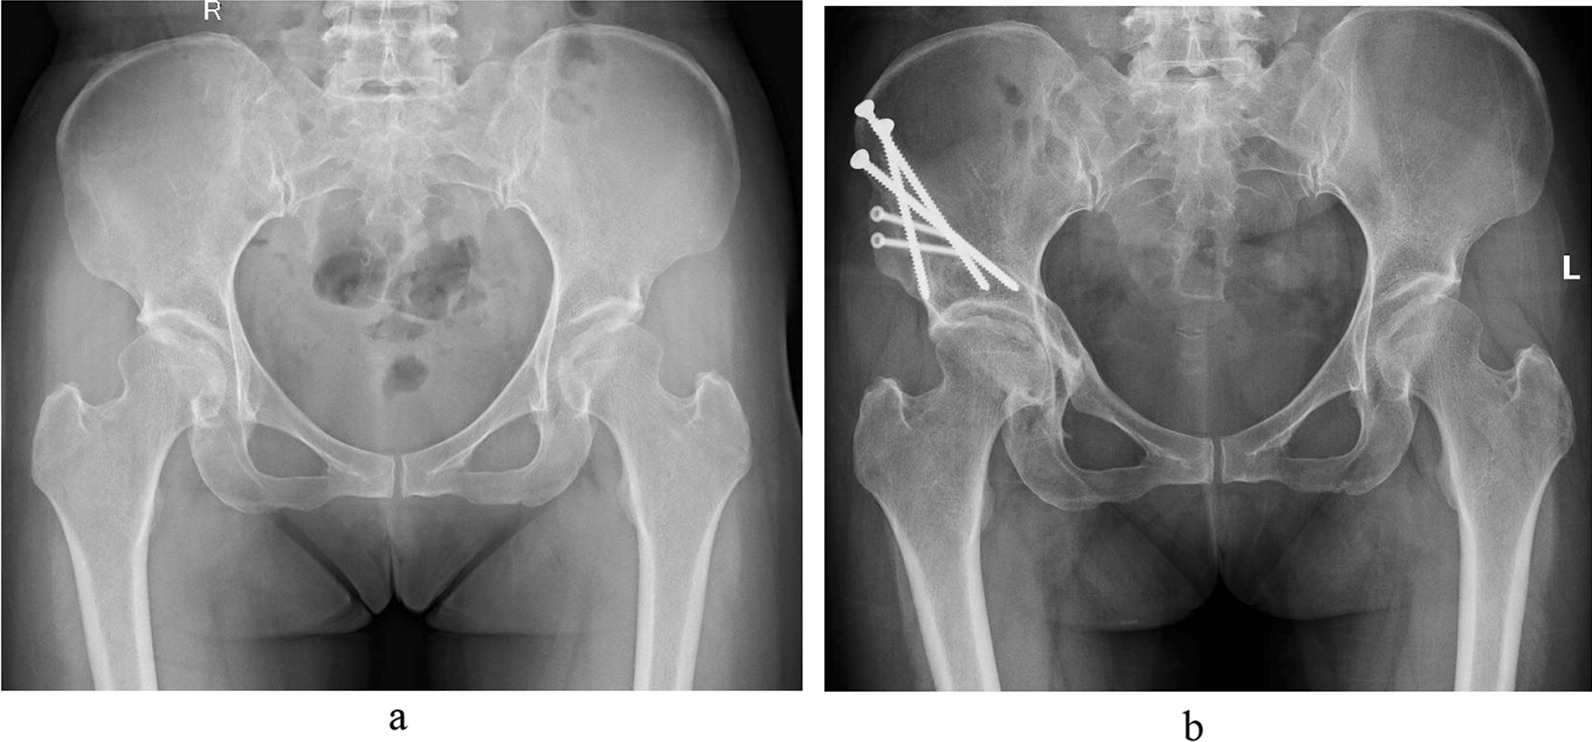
Female, 46 years. a Preoperative X-ray. b Postoperative X-ray. The preoperative LCEA was 24°, and the postoperative LCEA was adjusted to 55°. The preoperative HHS (iHOT-12) was 67 (58), and the postoperative HHS was 73 (70). The MCID of HHS (iHOT-12) was 6 (12)
